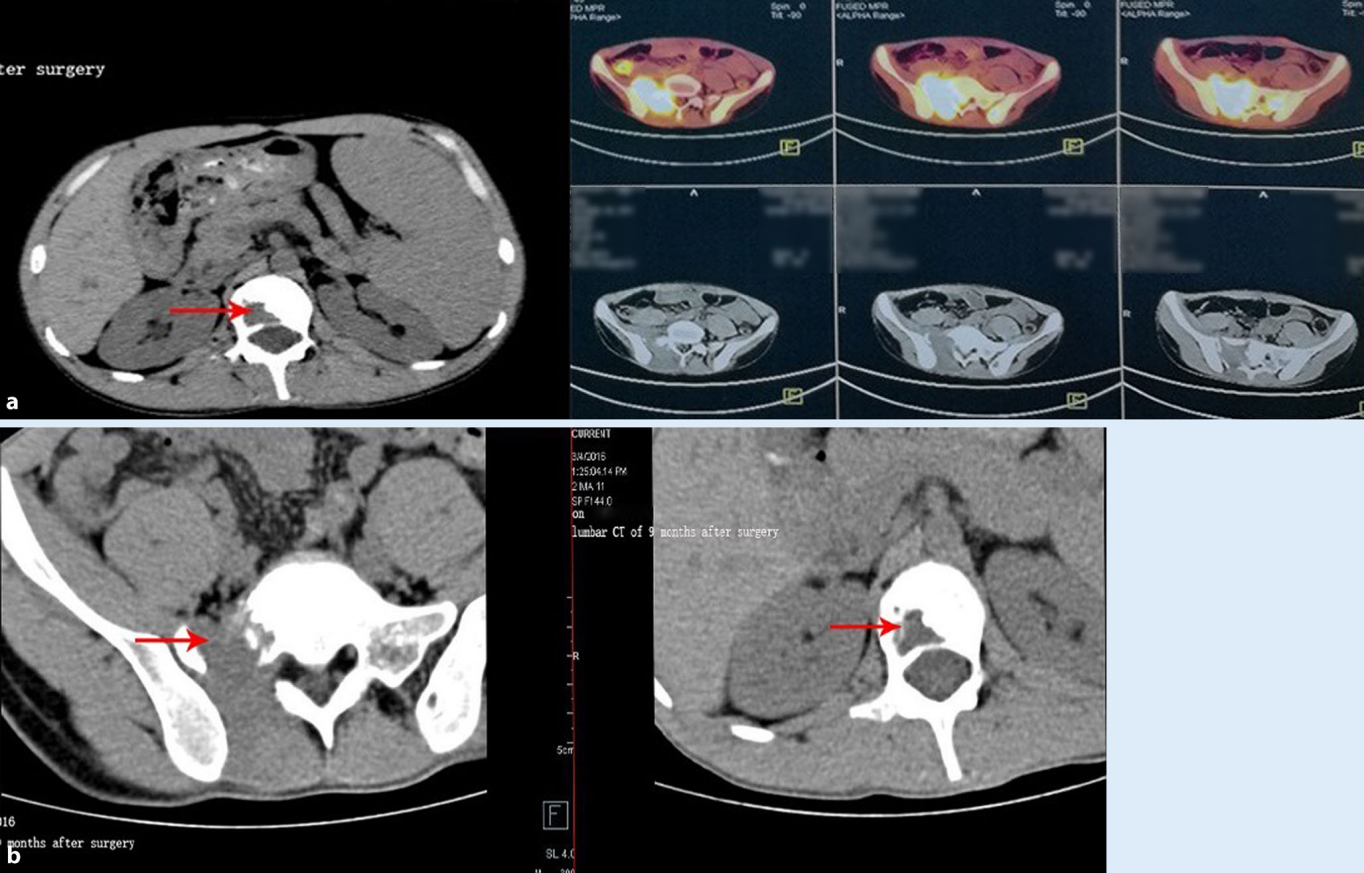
Computed tomography at a 3 months and b 9 months reveals no symptoms of recurrence and no changes in the lesions. *Red arrows* show improvement of lesions at 3‑month and 9‑month follow-up
